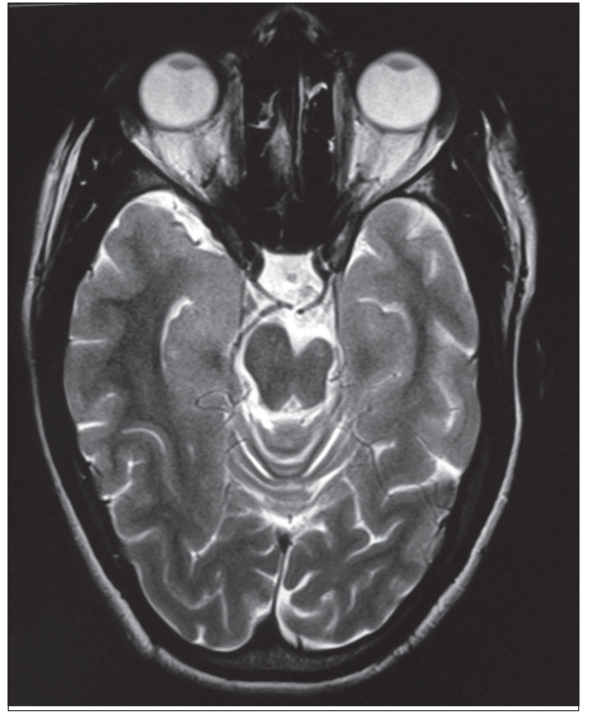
Axial T2-weighted image: Hypoplasia of left cerebral peduncle
